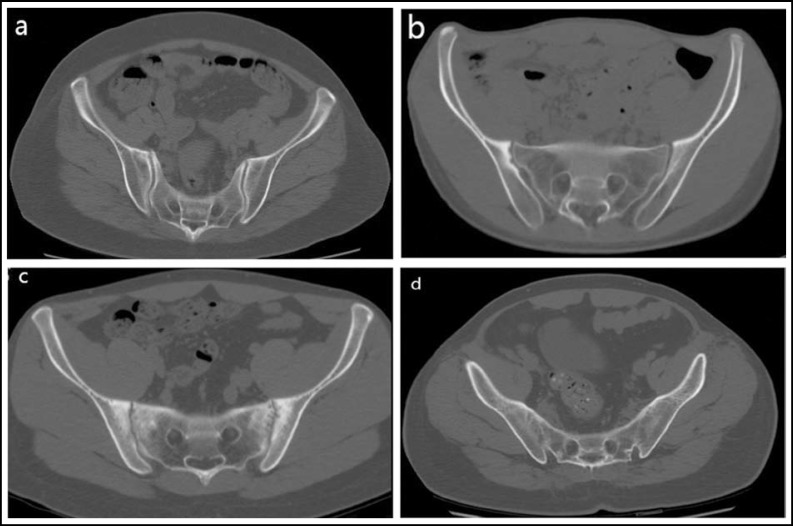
Grading of sacroiliitis is shown on the axial image of CT by using the bone window setting. (a) grade 1 shows suspicious changes, (b) grade 2 shows small localized areas with erosion or sclerosis, (c) grade 3 shows definite abnormality with erosions, sclerosis, joint space widening or narrowing, (d) grade 4 shows complete ankylosis
